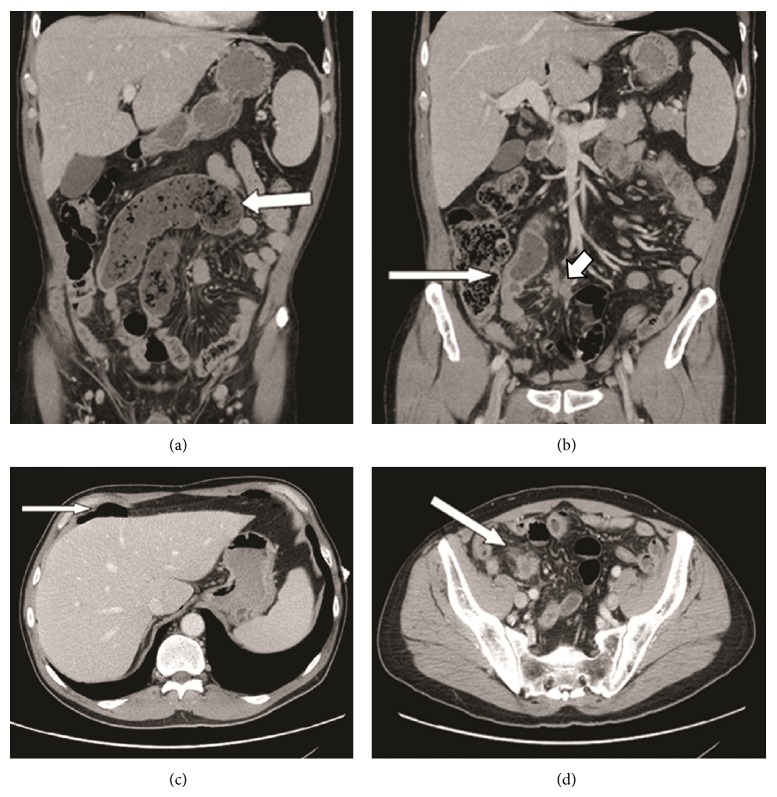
(a) Coronal contrast-enhanced CT image shows a markedly dilated fluid filled small bowel loop in the mid to right abdomen (*arrow*). There is evidence of stasis with small bowel fecalization sign. (b) Coronal contrast-enhanced CT image shows point of transition with associated irregularity and thickening of the bowel wall (*large arrow*). Note the abnormal enhancing lymph node (*small arrow*) in the adjacent small bowel mesentery (medially). (c) Axial contrast-enhanced CT image shows free intraperitoneal air below the ventral abdominal wall (*arrow*) in keeping with perforation. (d) Axial contrast-enhanced CT image shows a necrotic enhancing lymph node in the right lower quadrant (*arrow*).
